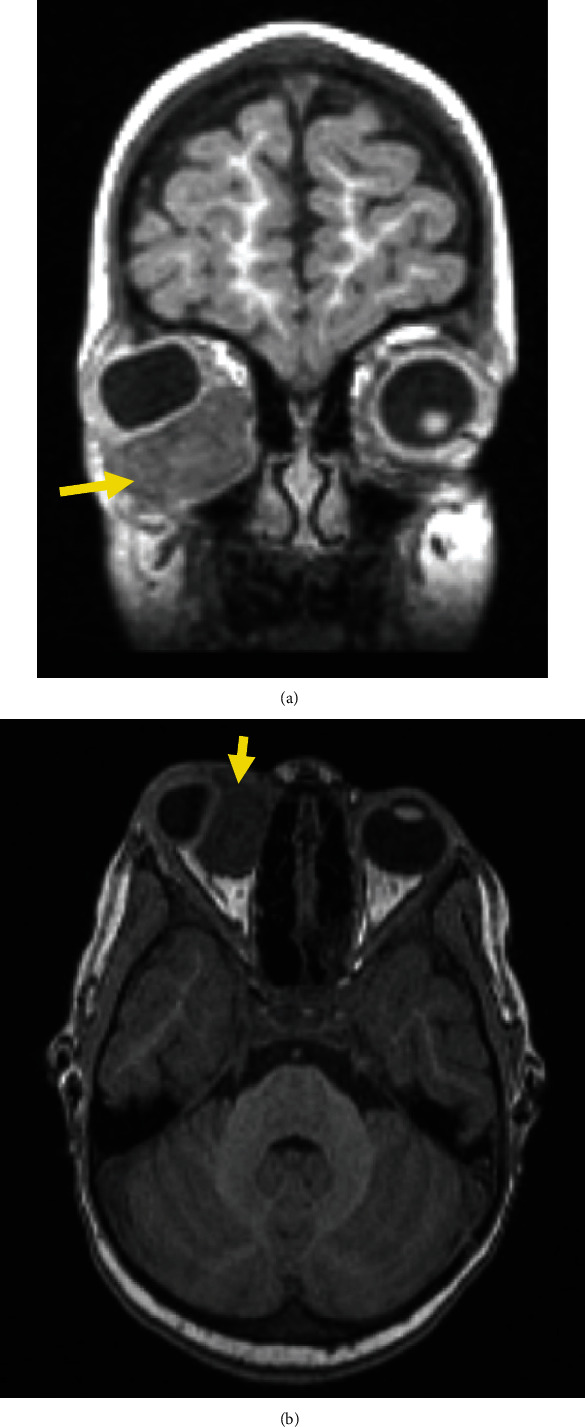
(a, b) MRI images demonstrate heterogeneously enhancing 2.7 cm extraconal mass lesion (yellow arrows), inferior to right globe and displacing it superolaterally.
